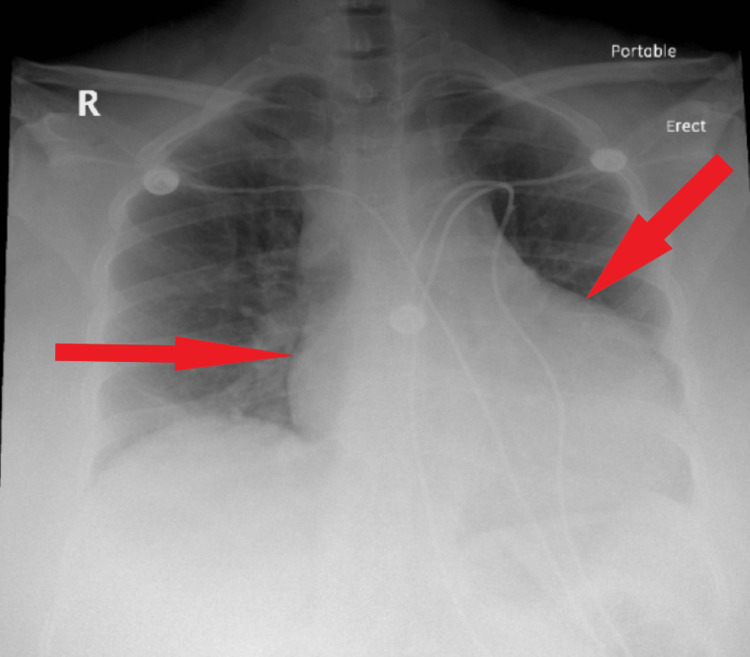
Follow-up chest X-ray posteroanterior view on the next day after admission The chest radiograph shows an enlarged cardiac silhouette, obscuring the lung hilum, indicative of pericardial effusion
